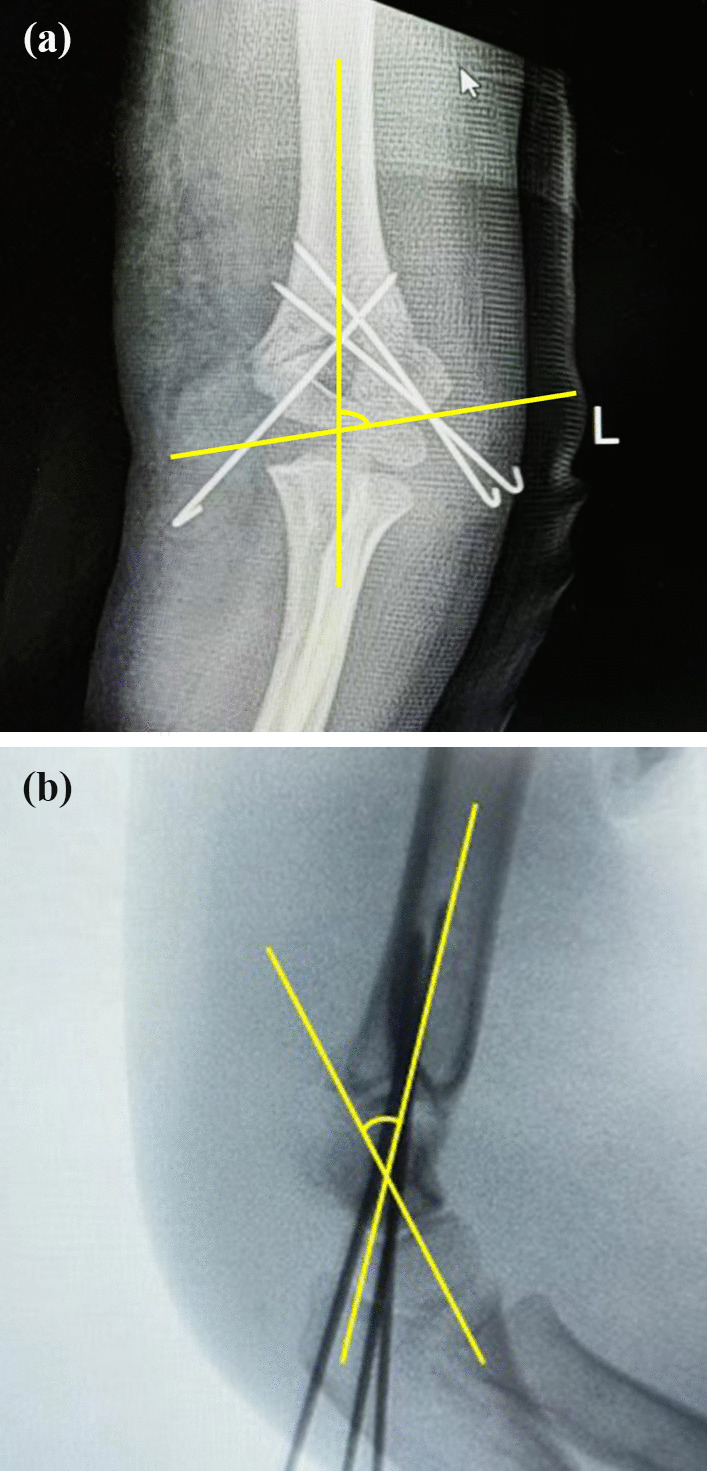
Measurement of the Baumann angle (a) and SCA (b) on the frontal and lateral X-rays, respectively
